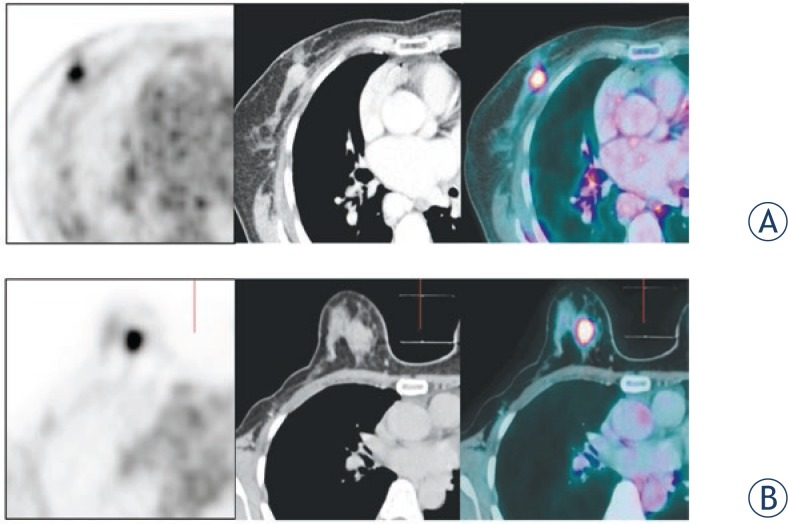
A woman with right breast cancer performed the supine-PET/CT and mammo-PET/CT. A The primary tumour was abutted on the chest wall in the supine-PET/CT. BThe tumour could be more clearly distinguished from the chest wall in the mammo-PET/CT.
